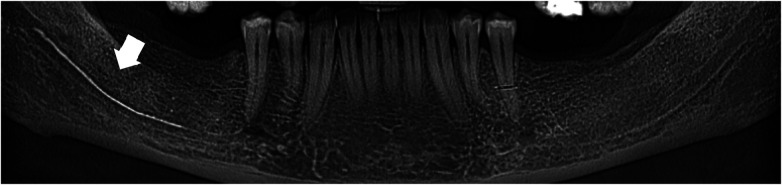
Coronal CBCT section taken after 3 years of follow-up revealed bone healing following extraction of the tooth 46, with persistence of the material in the IAN canal and partiel resorption.
